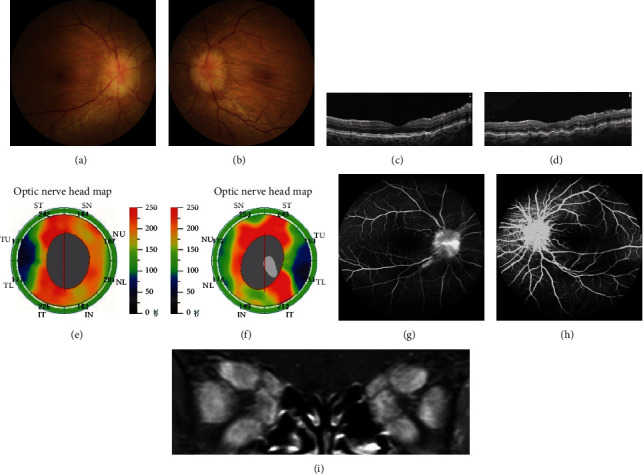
TAO patient a 50-year-old female who presented with bilateral CRFs and optic nerve edema. (a, b) The fundus photograph of bilateral CRFs with optic nerve edema. (c, d) The OCTA of bilateral CRFs in macula. (e, f) Bilateral CRF optic nerve edema of optic nerve head in the OCTA finding. (g, h) The bilateral CRF optic nerve edema of optic nerve head in the FFA finding. (i) MRI represents the crowded orbital apex and phlegmonosis from the enlarged extraocular muscles.
